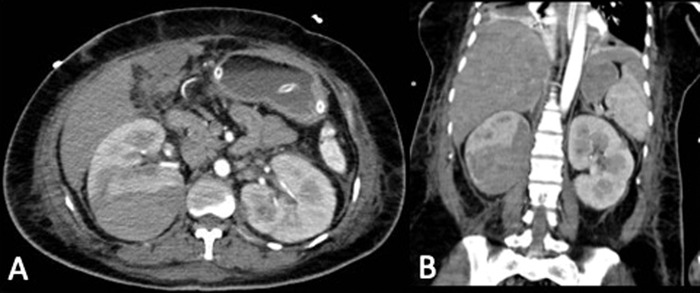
Abdominal-pelvic (A) transverse and (B) coronal computed tomography with contrast shows a perirenal hematoma with dimensions of 10 × 7.3 × 5.4 cm and a volume of 205 cc in the posterior region of the right kidney.
